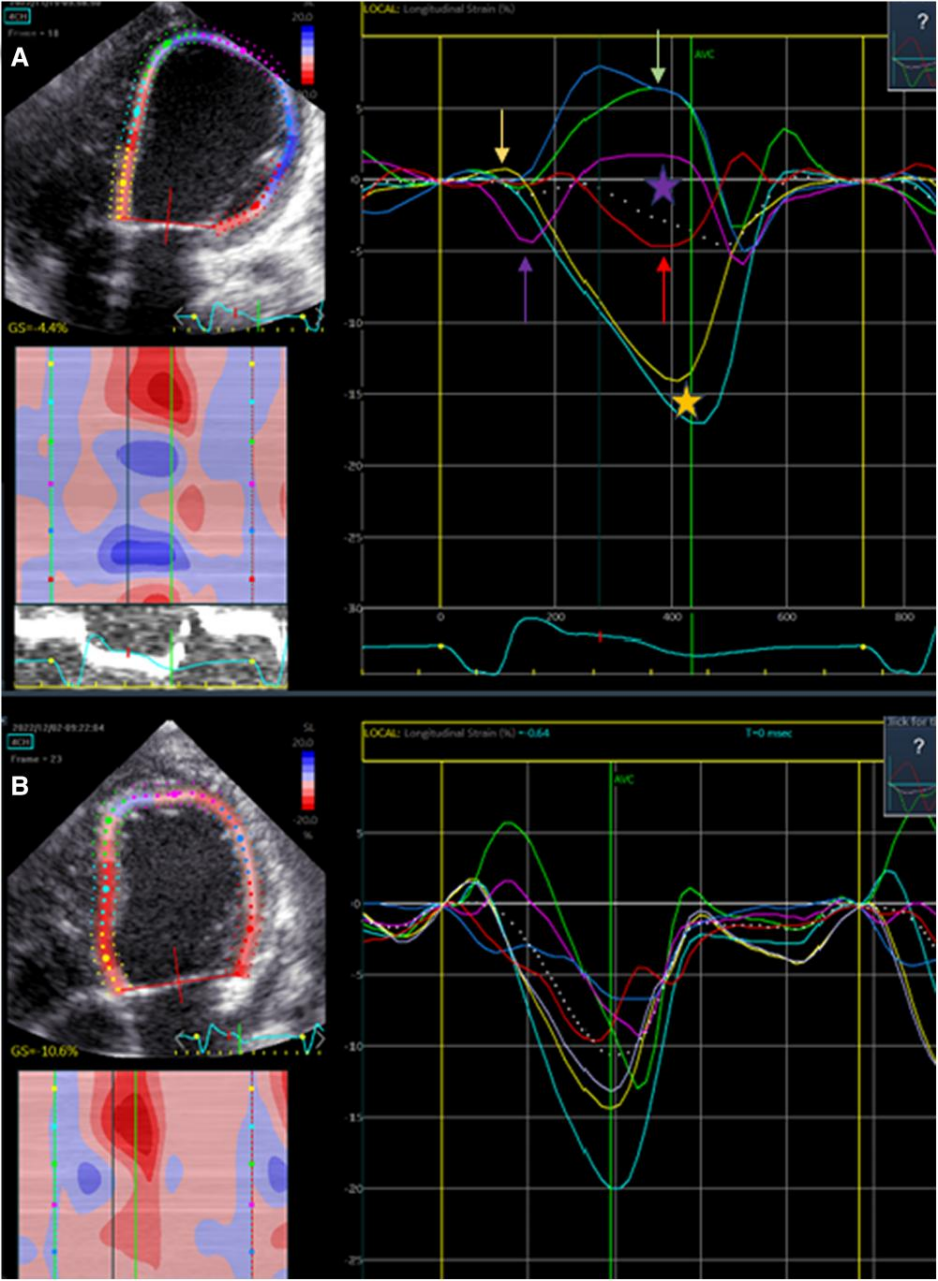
(*A*) Left ventricular (LV) systolic strain curve in the apical four-chamber view before CRT. The apex shows an early contraction (purple arrow) and minimal basal stretch (yellow arrow) with a late rebound stretch (green arrow and purple star) and a basal late contraction (red arrow and yellow star). (*B*) LV systolic strain curve in the apical four-chamber view after CRT with extra lead placement on the LV basal free wall. The LV contracts clearly in a more homogeneous manner with an increased apical to basal synchrony.
